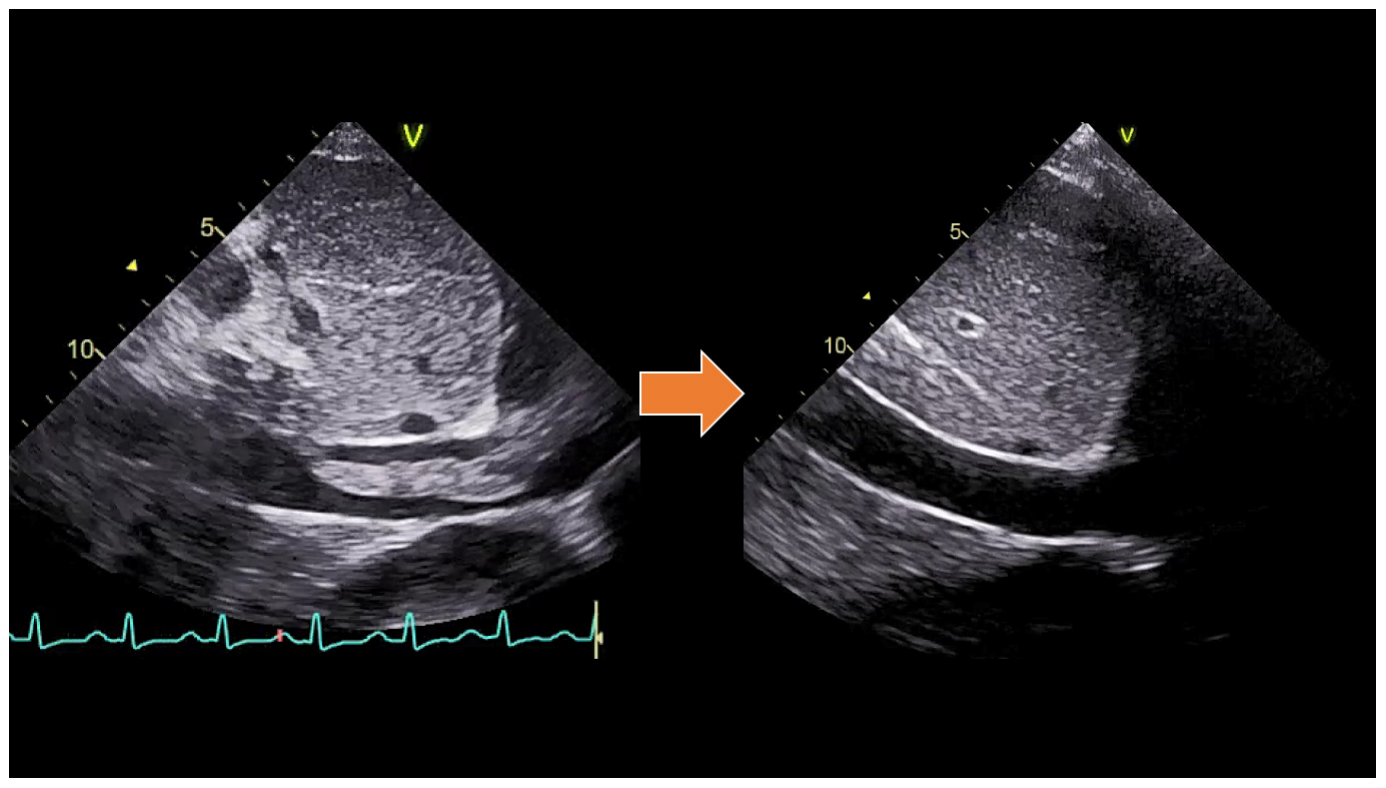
Transthoracic echocardiogram (TTE) comparison showing the position of the thrombus moving from the inferior vena cava towards the right atrium.
